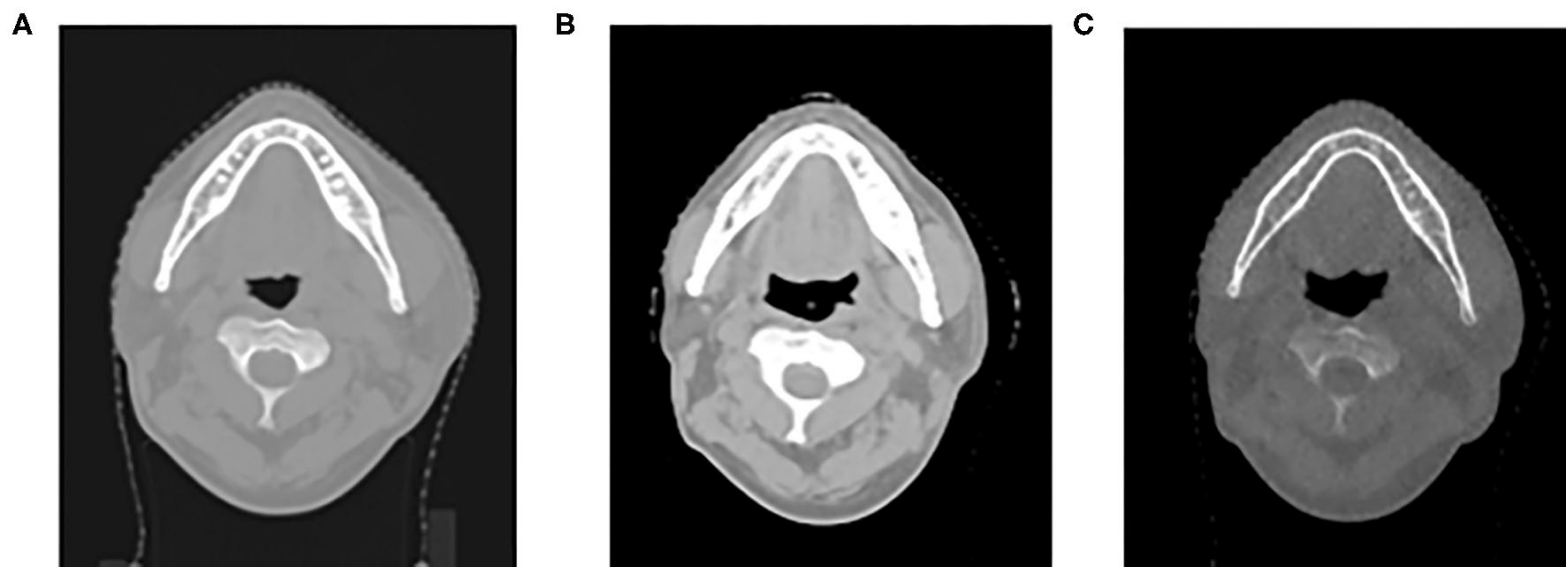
Planning CT (A), rescanned CT (B), and corresponding megavoltage cone-beam CT (MVCBCT) image on the same day of rescanned CT (C) of one example patient.
